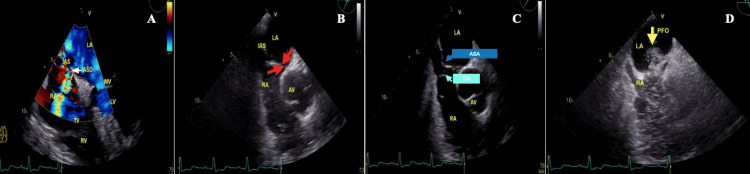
The echocardiographic images of the patient Fourth-degree four-space image, color Doppler shows a transition from LA to RA. Black arrow with a yellow frame, atrial septal defect (ASD) (A); 60-degree aortic valve level. Black arrow with a yellow frame, patent foramen ovale (PFO) (B); aortic valve level. Black arrow with a yellow frame, atrial septal aneurysm (ASA) and Chiari network (CN) (C). Black arrow with a yellow frame, a transition of contrast from the right atrium to the left atrium via PFO (D). RA, right atrium; TV, tricuspid valve; RV, right ventricle; LA, left atrium; MV, mitral valve; LV, left ventricle; IAS, interatrial septum; AV, aortic valve
